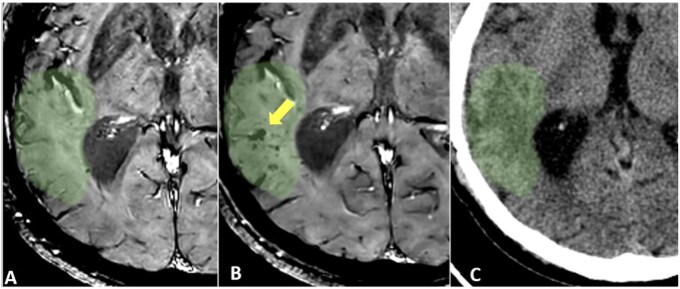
Pre and postsonication imaging in a patient during the first cycle. A-B SWI sequences at pre-treatment MRI (A) and 1-day FU (B) reveal the postoperative appearance of black foci in the sonicated cerebral tissue. (C) 1-day postoperative CT shows the absence of detectable hemorrhagic areas. The green area identify the treated volume, and the red arrow indicates the black spot.
